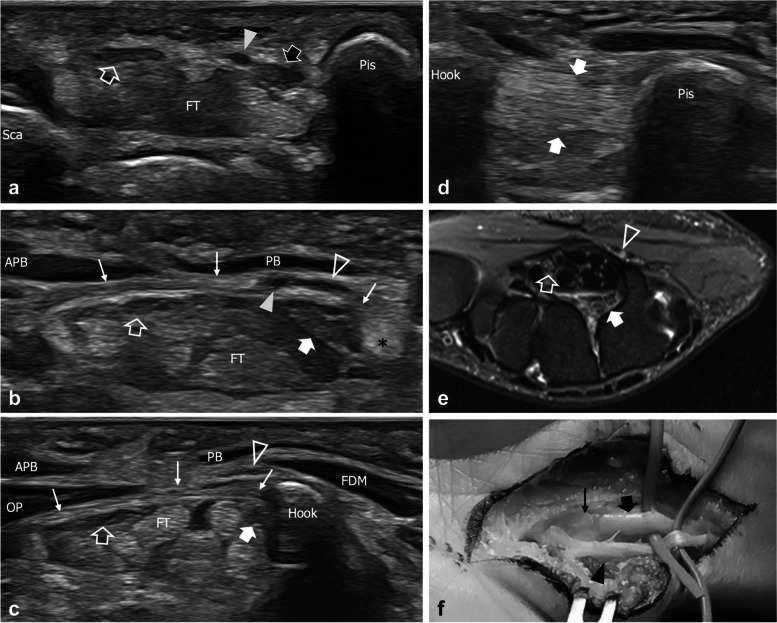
Level one entrapment neuropathy in a 29-year-old woman with progressive wasting of the interossei. a, b, c Consecutive short-axis 22–8-MHz US images from proximal to distal demonstrate a hypoechoic and swollen ulnar nerve (black arrow) at the proximal part of the Guyon canal, on the radial side of the pisiform (Pis). Distal to this level, the deep branch (white arrow) appears running in an anomalous position inside the carpal tunnel underneath the flexor retinaculum (thin arrows), on the radial side of a hypertrophied pisohamate ligament (asterisk) and, more distal, of the hamate hook, whereas the superficial branch (outlined arrowhead) keeps on running along the regular path on the side of the ulnar artery (arrowhead). Note the edematous changes and the swollen appearance of the deep branch compared to the regular median nerve (outlined arrow). Sca, scaphoid; FT, flexor tendons; APB, abductor pollicis brevis; PB, palmaris brevis; OP, opponens pollicis; FDM, flexor digiti minimi. d Long axis 22-8MHz US image better demonstrates the thickened pisohamate ligament (arrow). e Transverse turbo Spin Echo T2-weighted MRI scan with fat saturation shows the anomalous position of the deep branch (arrow) inside the carpal tunnel on the radial side of the hamate hook. The superficial branch (arrowhead) is normally positioned over the superficial aspect of the carpal ligament. Outlined arrow, median nerve. f The intraoperative picture confirms the swollen appearance of the deep branch (black arrow) before it engages the carpal tunnel underneath the flexor retinaculum (thin arrow). Arrowhead, superficial branch
